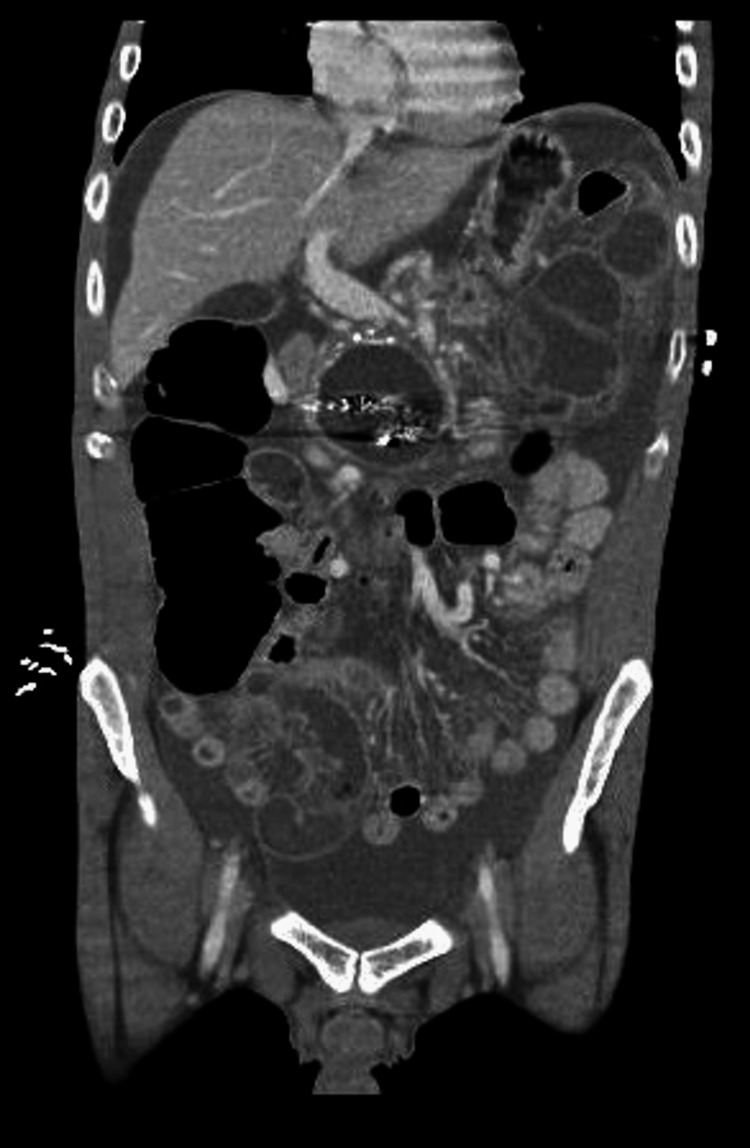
Computed tomography showing mild dilation of the proximal colon, concerning for ileus
